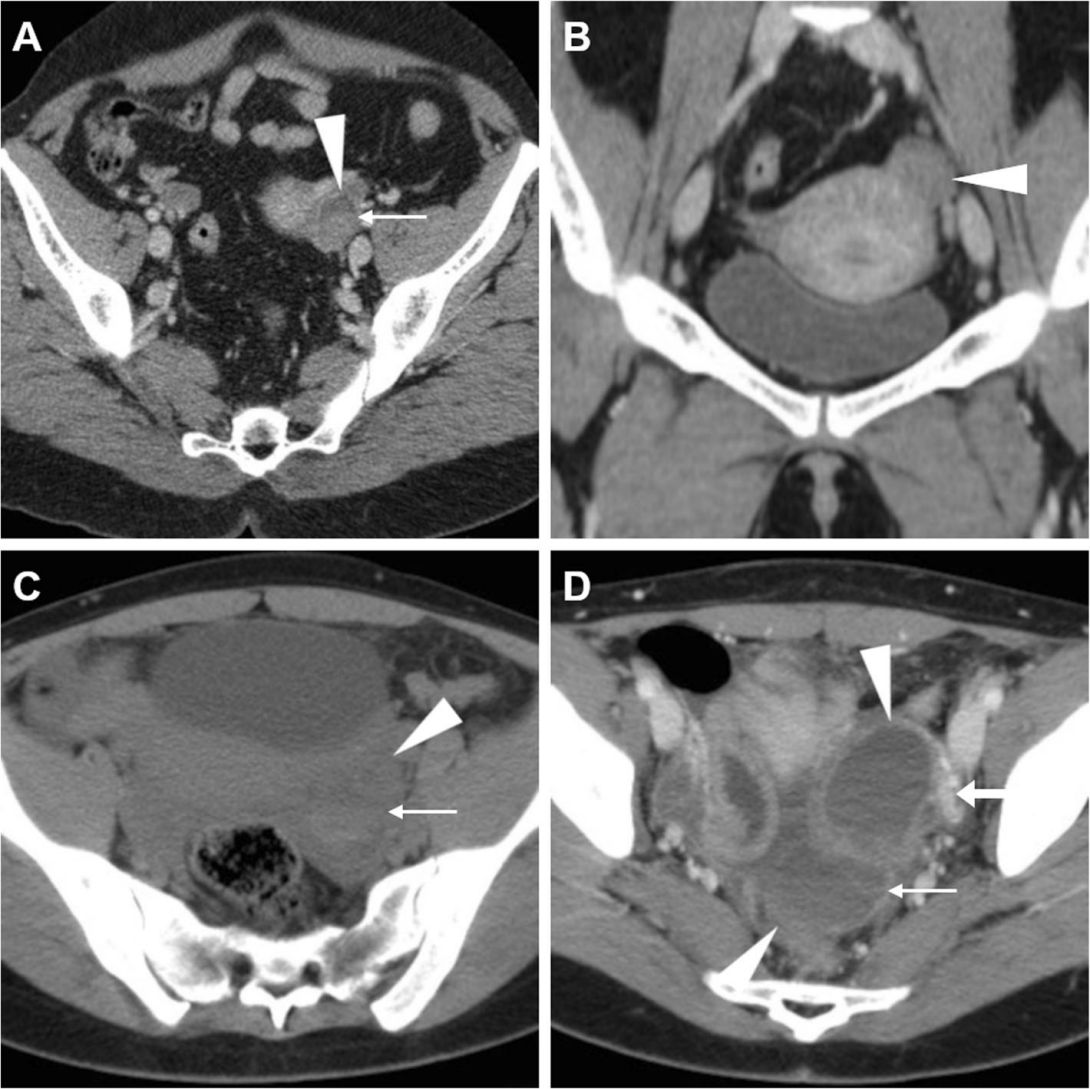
Two CT cases of haemorrhagic ovarian cysts. a, b The normal-sized left ovary (arrowheads) show mixed appearance with dependent high attenuation and fluid-fluid level (thin arrow in a) consistent with haemorrhagic corpus luteum. c, d Unenhanced (c) and post-contrast (d) images show a larger, bilocular adnexal lesion (arrowheads) with thin regular peripheral enhancement and dependent hyperattenuation (thin arrows). Note the ovarian vessels (arrow in d)
